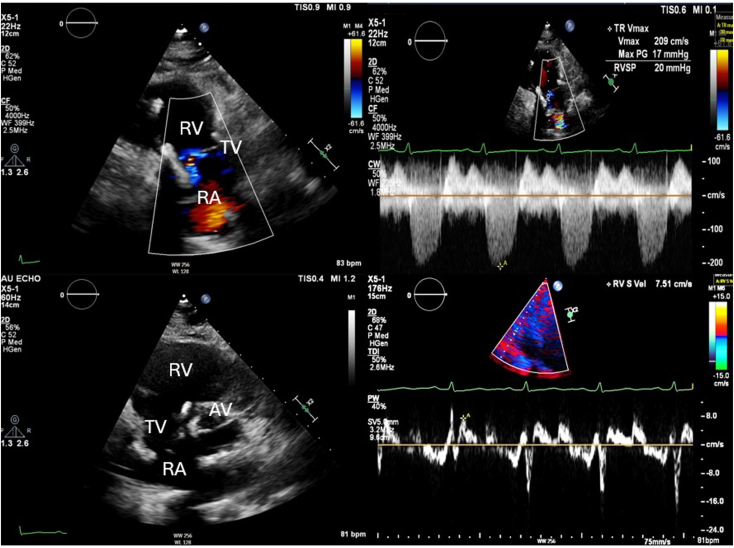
Postoperative two-dimensional TTE, parasternal long-axis right ventricular inflow systolic view with color-flow Doppler (*top left*) and continuous-wave Doppler (*top right*), right ventricular inflow-outflow short-axis systolic view (*bottom left*), and tissue Doppler spectral display of the tricuspid annulus (*bottom right*), demonstrates a dilated RV with moderate residual TR and decreased right ventricular systolic function.
